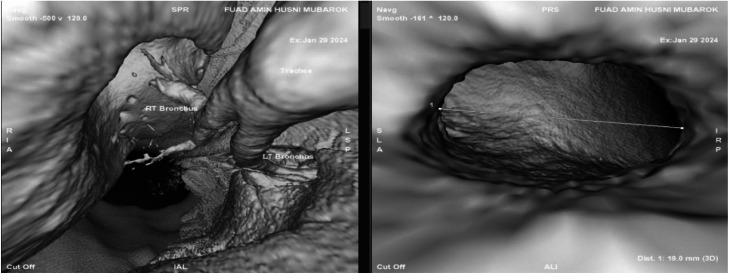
CT virtual bronchoscopy revealed a fistulous tract in the right secondary bronchus measuring 19 mm.
